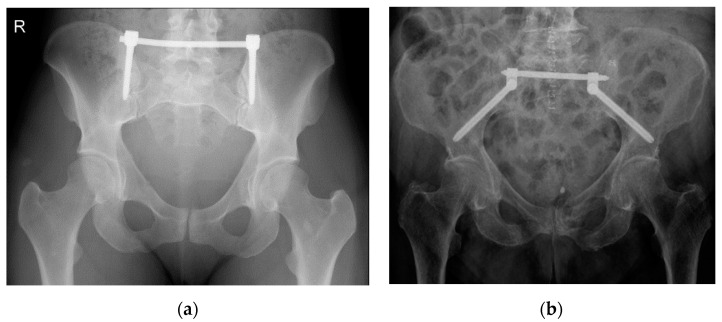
X-ray of a transiliac internal fixator (TIFI)-osteosynthesis in cranio-caudal (a) and dorso-ventral (b) orientation.
